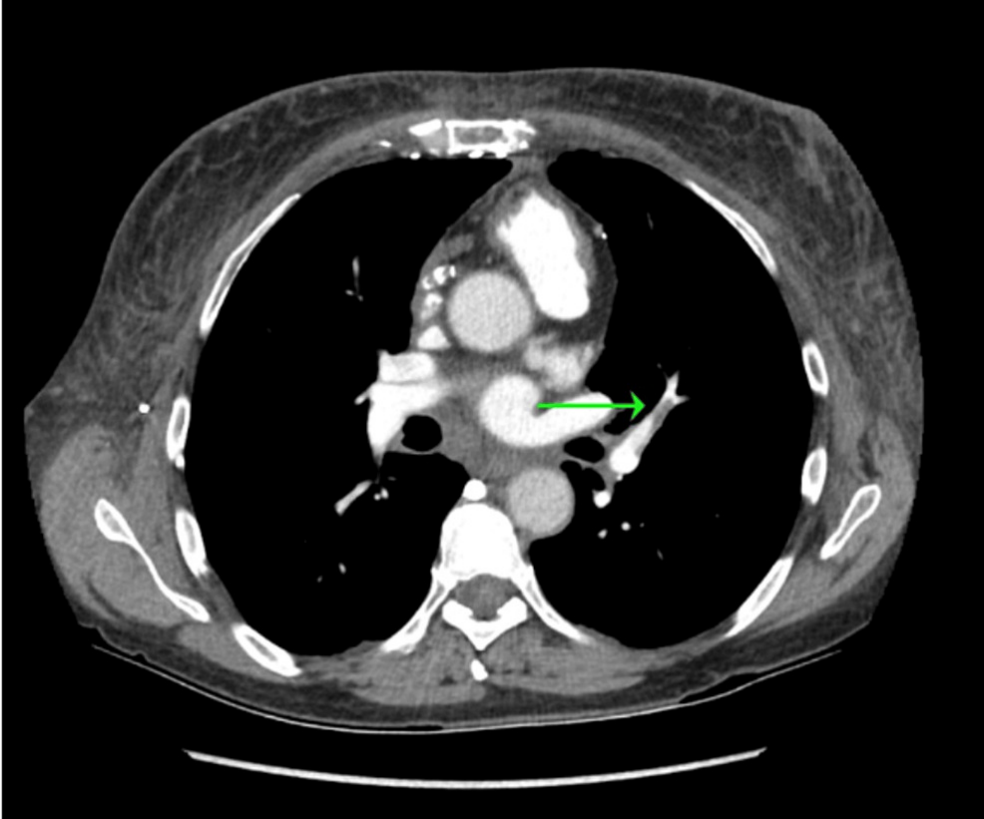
CTPA (axial view) showing subsegmental pulmonary emboli CTPA: computed tomography pulmonary angiography
